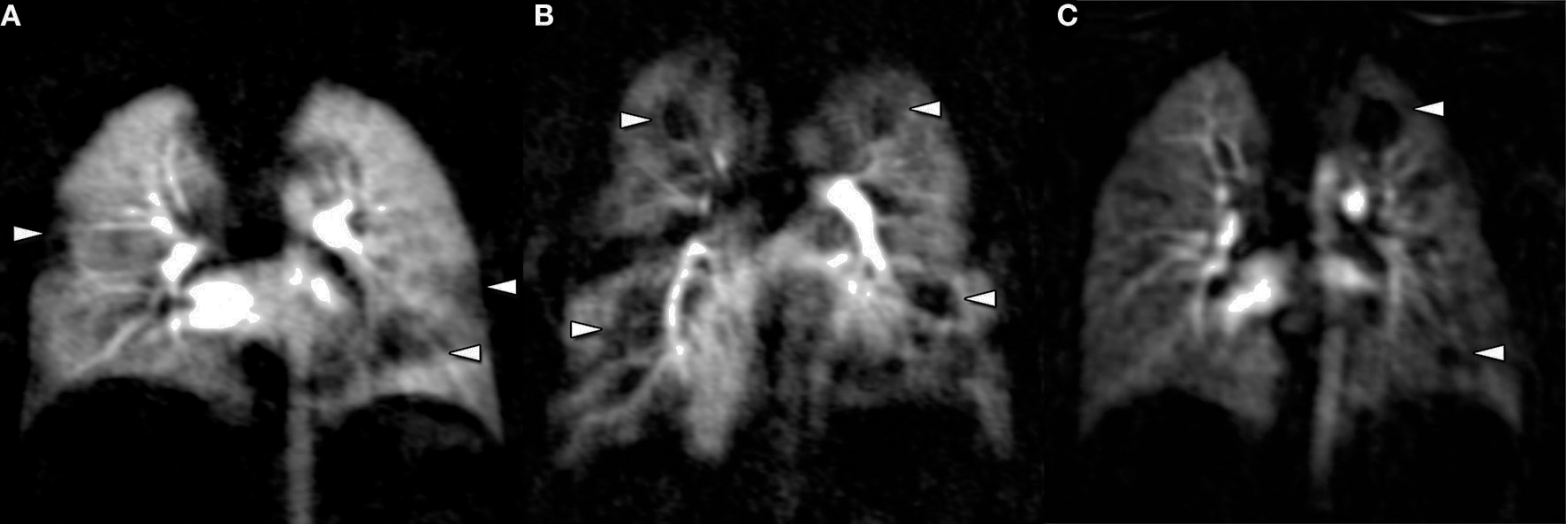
Perfusion MRI is a ready-to-use technique to study functional impairment in pediatric lung disease, based on gadolinium enhancement. Perfusion abnormalities can be found in conjunction with structural lung disease in cystic fibrosis (A), Langerhans cell histiocytosis (B), or bronchopulmonary dysplasia (C). Of note, perfusion abnormalities are non-specific and must be reviewed together with morphological imaging studies.
